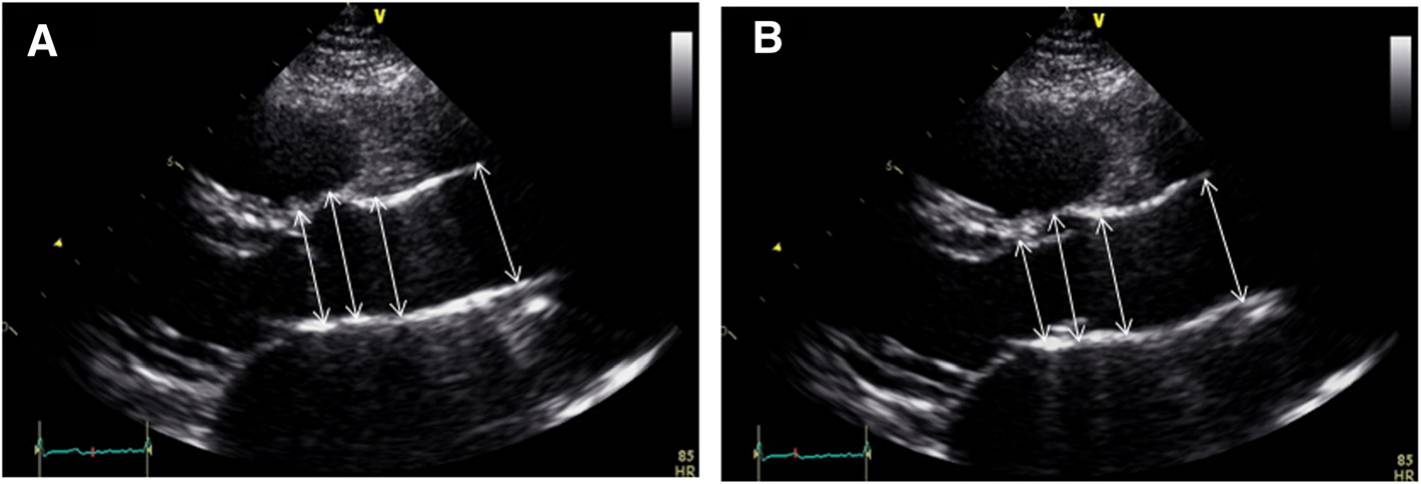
Representative case of measurement of aortic diameter by (A) 2005 American Society of Echocardiography (2005 ASE) chamber quantification guidelines and (B) 2010 ASE pediatric measurement guidelines (2010 Pediatric).
